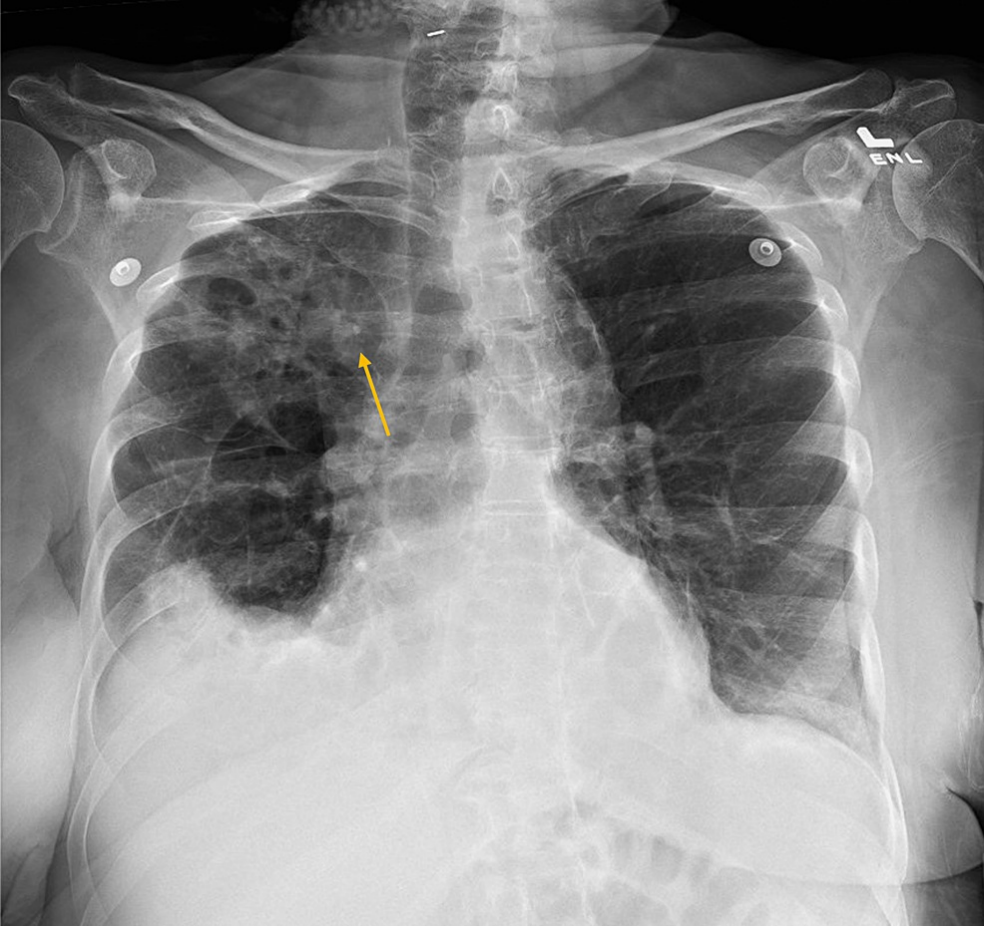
Chest X-ray (anterior to posterior view) The arrow shows right upper lobe consolidation.
